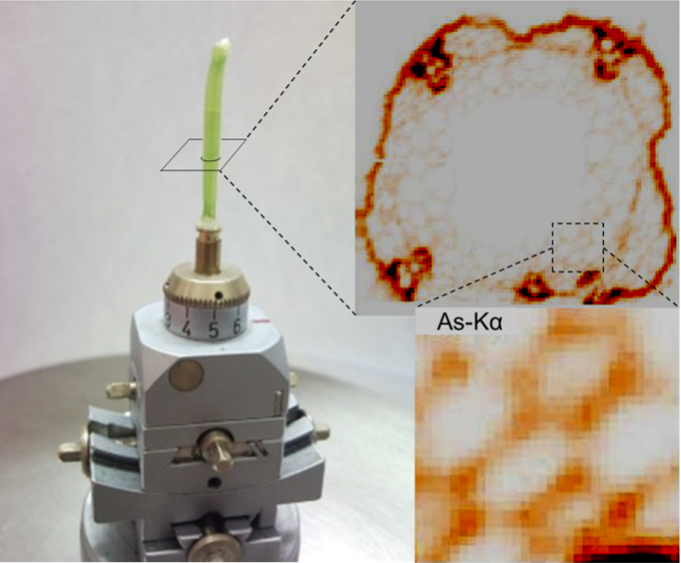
An optimized micro-X-ray
